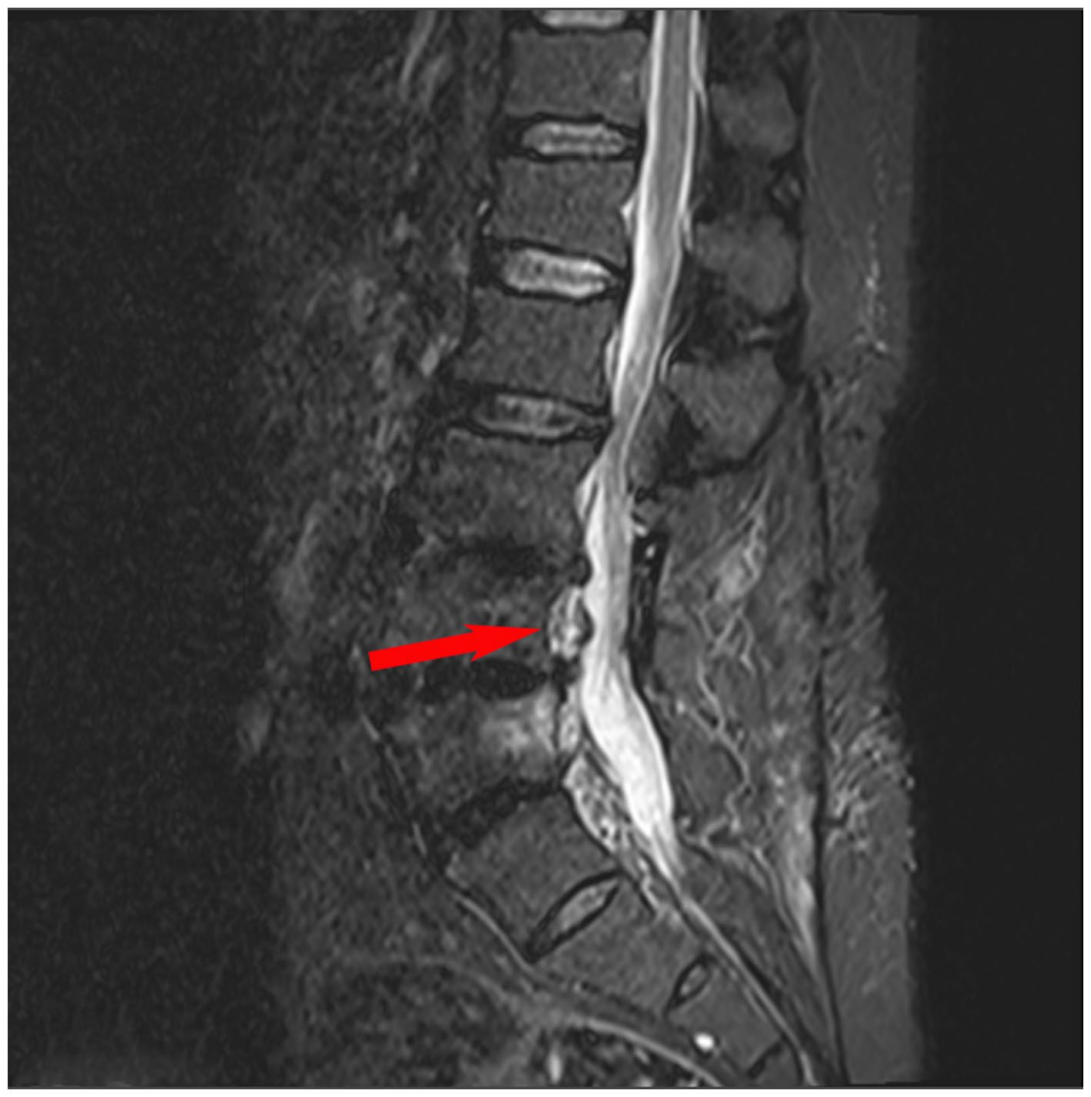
Sagittal magnetic resonance imaging (MRI) showing infection in the L3–L5 vertebrae with an intraspinal abscess of the patient before treatment.
